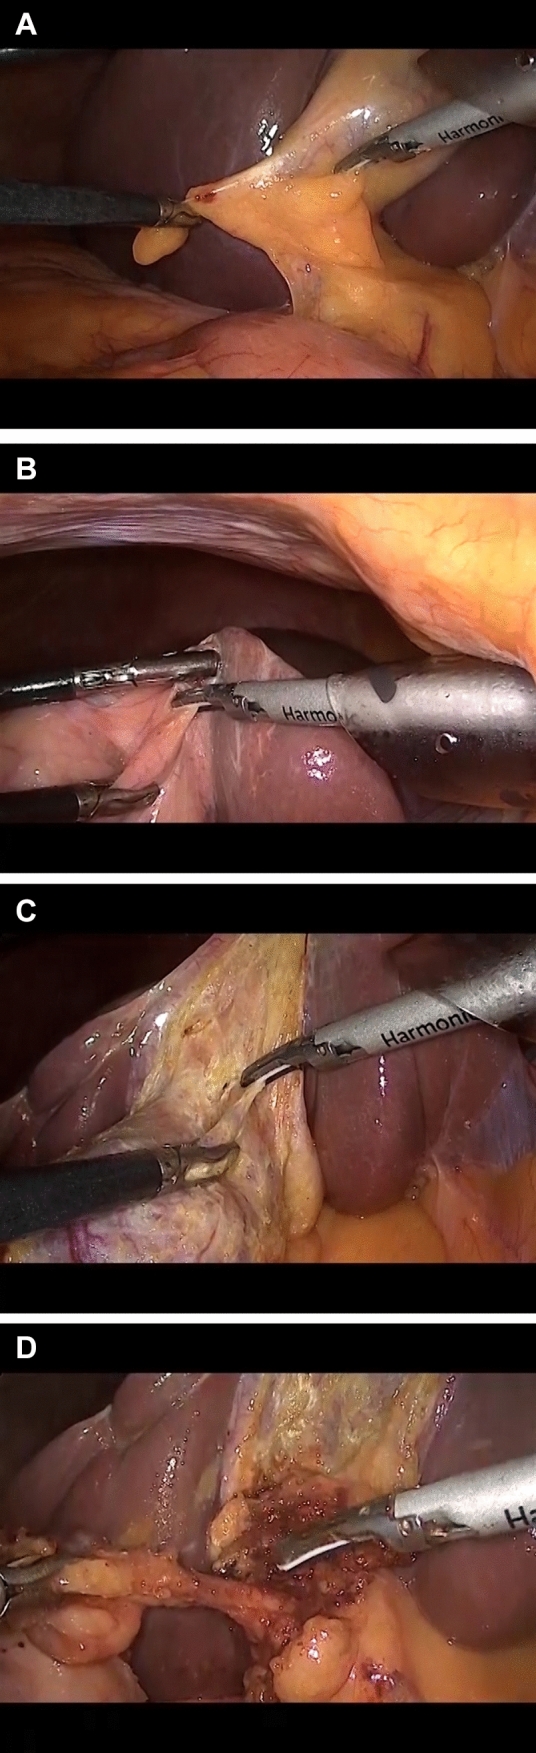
A–D The ultrasonic fundus-first dissection. A The dissection starts by marking the peritoneum where the surgeon estimates that the gallbladder continues into the cystic duct. B The assistant places a grasper on the peritoneum between the top of the gallbladder and the liver. The dissection then begins, and the correct space is identified. C The dissection continues, alternating on the medial and lateral sides, until the already-defined marking is reached. D The artery and cystic duct in the triangle of Calot are cleared and visualized. A cholangiography is performed, and the artery and cystic duct are divided by using at least two proximal clips
